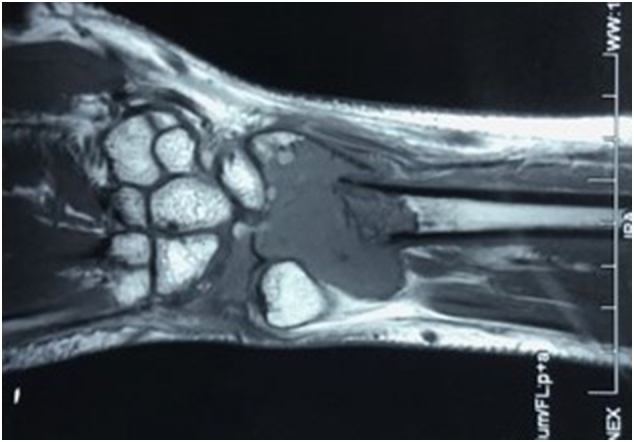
MRI scan of the distal radius showing a Campanacci grade 3 GCT at the distal radius with a pathological fracture.
